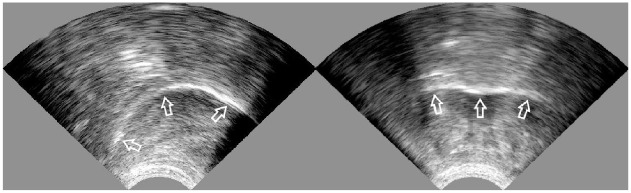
Sagittal (on the left; the front of the tongue is on the right side of the image) and coronal (on the right) ultrasound tongue images. The arrows mark the tongue surface at the bottom edge of the bright curve.
